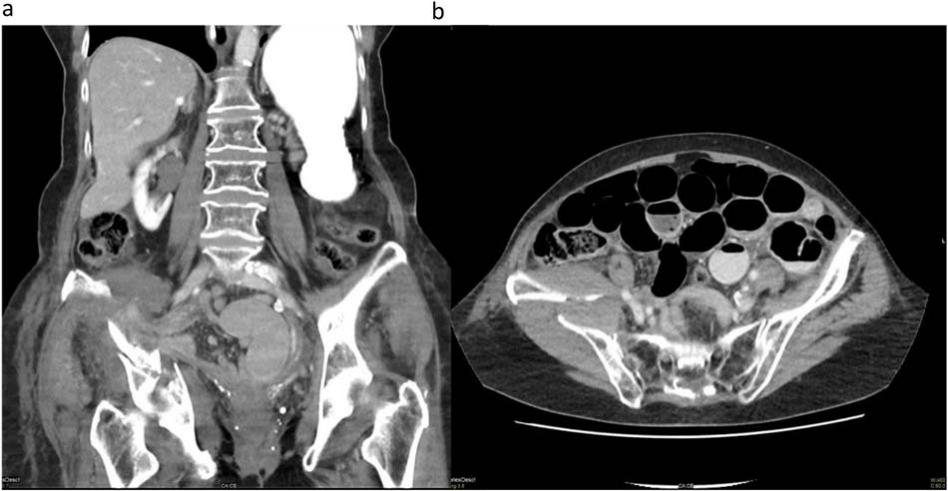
(a) Coronal CT image demonstrating the acetabulum fracture on the right side with herniated mesenteric fat tissue and collapsed small bowel loops (white arrow). (b) Transverse CT image demonstrating dilated small bowel loops and a distinct transition point (white arrow) on the right side close to the acetabulum fracture and a haematoma.
